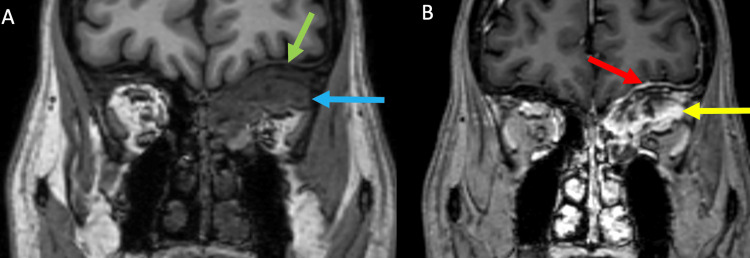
MRI brain without and with contrast: Pre-contrast T1 weighted image (A) shows replacement of the marrow (green arrow), invasion and obliteration of the superonasal extra-conal orbital fat (blue arrow), and loss of fat plane to the superior rectus muscle. After the administration of intravenous contrast (B), the mass shows heterogeneous enhancement (yellow arrow). Enhancement of the dura (red arrow) may be reactive inflammation or early tumor infiltration.
